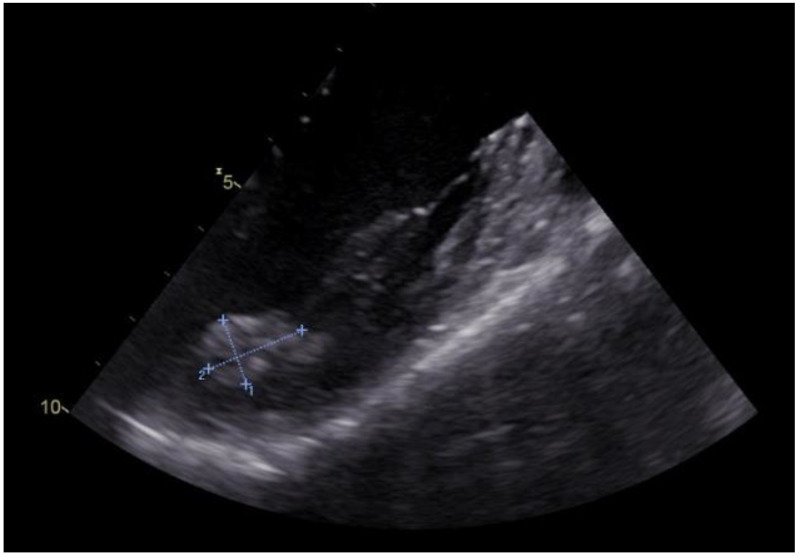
Two-dimensional transesophageal echocardiography of the tumour in the left ventricle and papillary muscle at the base of the anterolateral papillary muscle (orange arrow, tumour; blue arrow, anterolateral papillary muscle).
